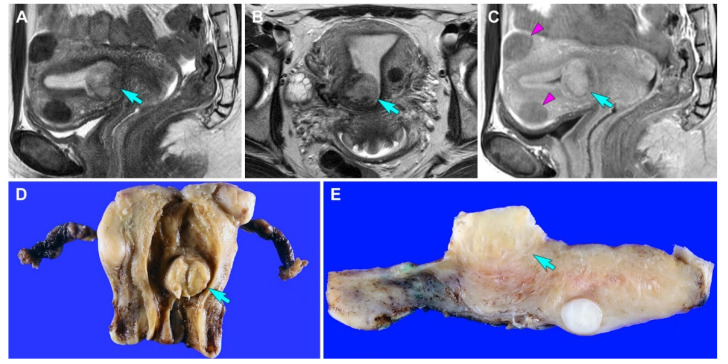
Imaging and gross findings (case 1). (A,B) T2-weighted (A) sagittal and (B) axial magnetic resonance imaging (MRI) reveals a relatively well-circumscribed mass in the uterus (blue arrows). (C) Contrast-enhanced T1-weighted turbo spin-echo sagittal MRI reveals that the tumor appears to involve the superficial myometrium. The signal intensity of the uterine mass (blue arrow) is higher than that of intramural leiomyomas (purple arrowheads). (D) Grossly, a well-circumscribed mass (blue arrow) is located in the uterine corpus. (E) The cut section shows an exophytic mass (blue arrow) that appears to invade the superficial myometrium.
